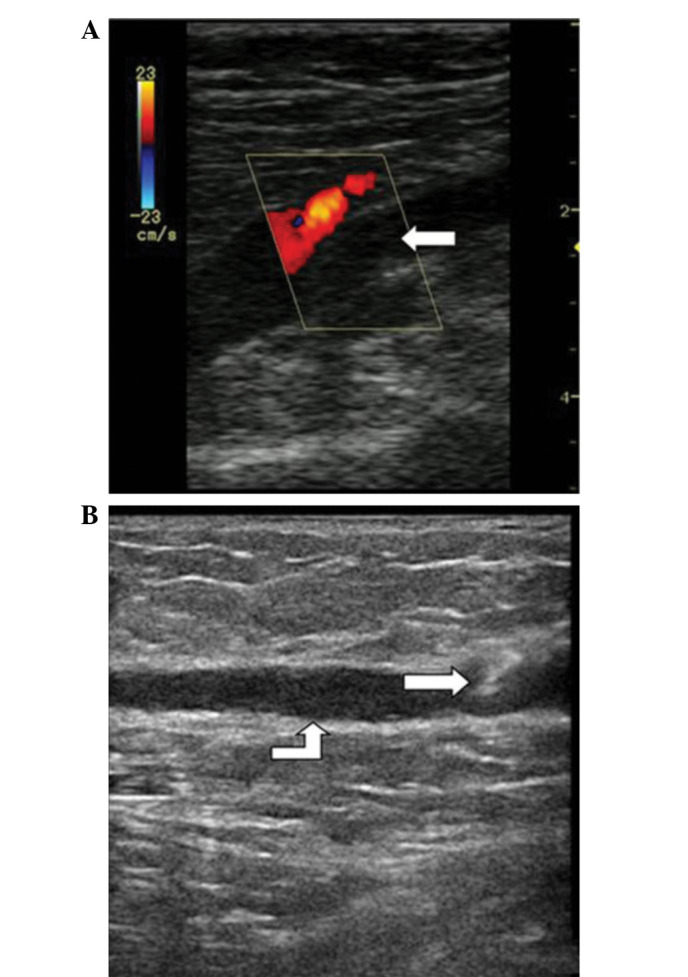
Color Doppler ultrasound of VCF placement. (A) deep vein thrombus (arrow); (B) puncture needle (straight arrow) and GSV (bent arrow). VCF, vena cava filter; GSV, great saphenous vein.
